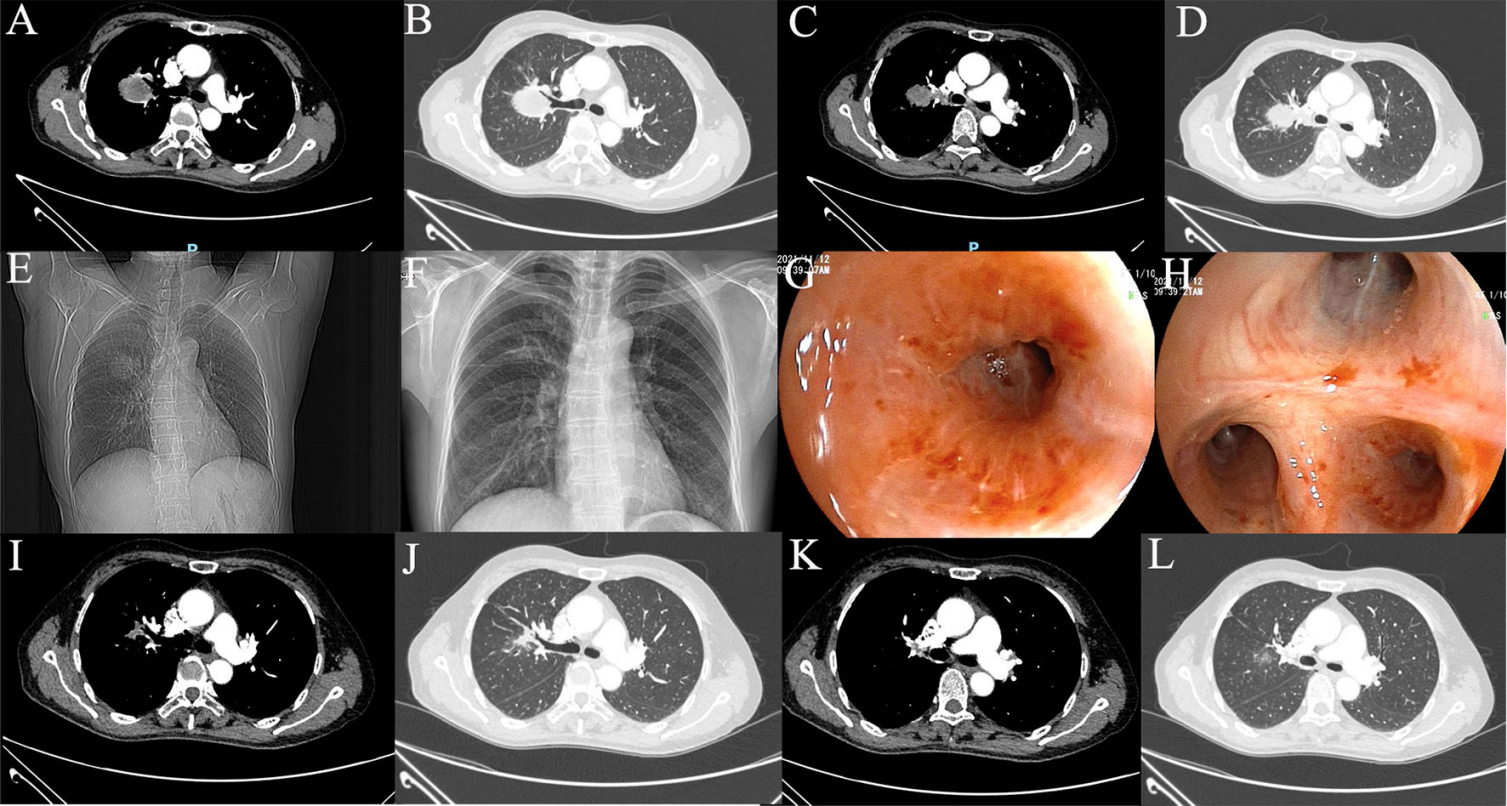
Imaging examination. (A–D) Preoperative CT scan; (E) Preoperative DR image; (F) DR image after one cycle of neoadjuvant therapy; (G, H) Preoperative fiberoptic bronchoscopy image; (I–L) CT image after 2 cycles of neoadjuvant therapy.
